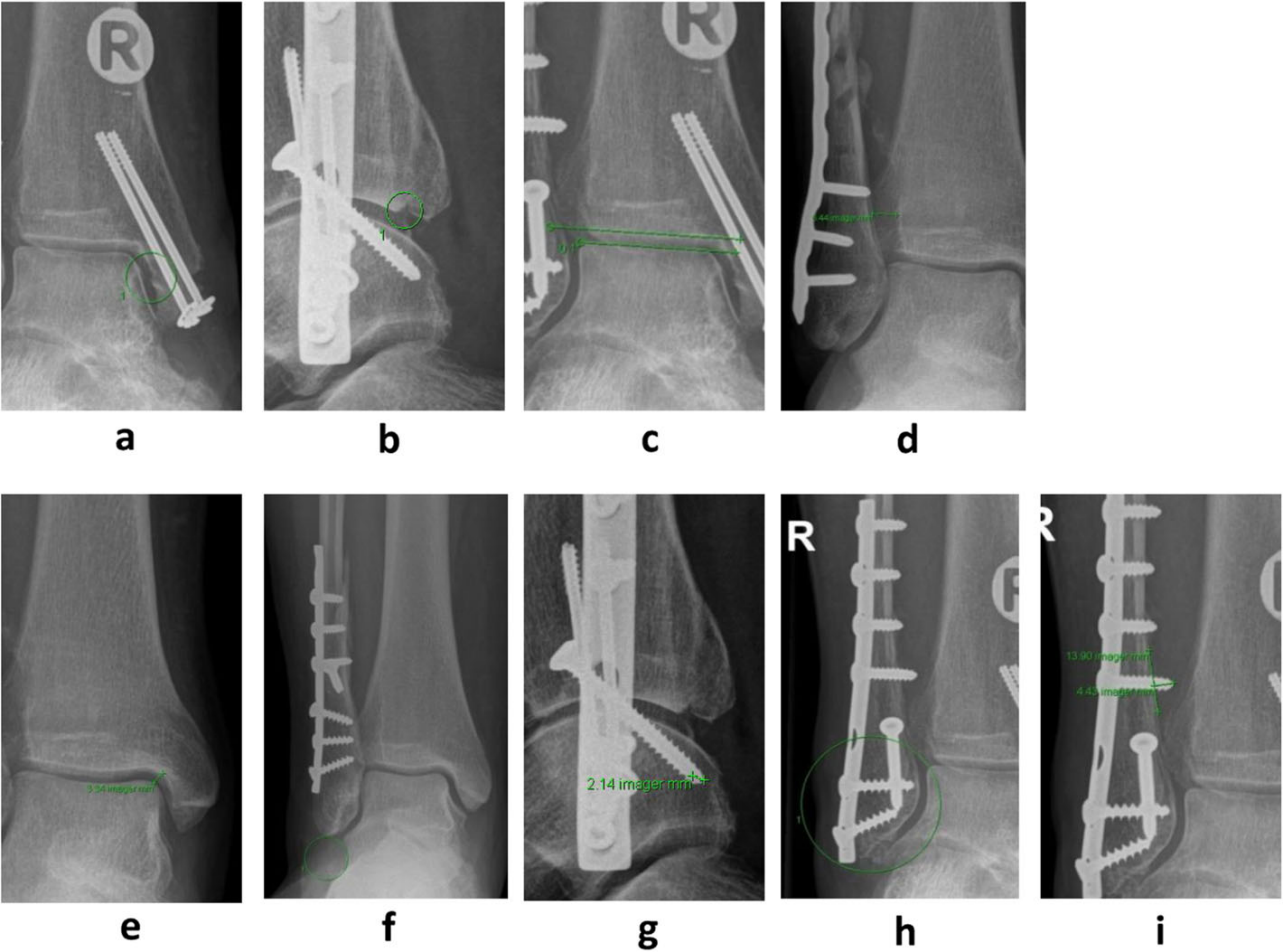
Fracture reduction criteria measured on post-operative X-ray 3 months after ankle surgery. 2a Medial step-off in mortise view. 2b Posterior step-off: only included if more than 20% of the articulating surface was affected in lateral view. 2c Tibiotalar tilt: measured in mortise view as the angle between the tibial plafond and the talar joint line. 2d Tibiofibular overlap: defined as the distance between the medial edge of the fibula and incisura fibularis and measured 10 mm proximal to the tibial plafond in mortise view. 2e Oblique medial clear space: measured between the inferior-medial corner of the tibial plafond and the superior-medial corner of the talus in mortise view. 2f Dime sign: fibula is shortened and reported as “positive” dime sign if the “ball” is broken in mortise view. 2g Lag screw surpassing the bone cortex (bicortical): the distance was measured perpendicular to the cortical line. 2h Distal fibular screws: defined as “positive” if any of the 3 distal screws was bicortical in any projection. 2i Proximal fibular screws: the distance was measured perpendicular to the cortical line of the most penetrating screw in any projection. All measurements were reported in mm or positive/negative
